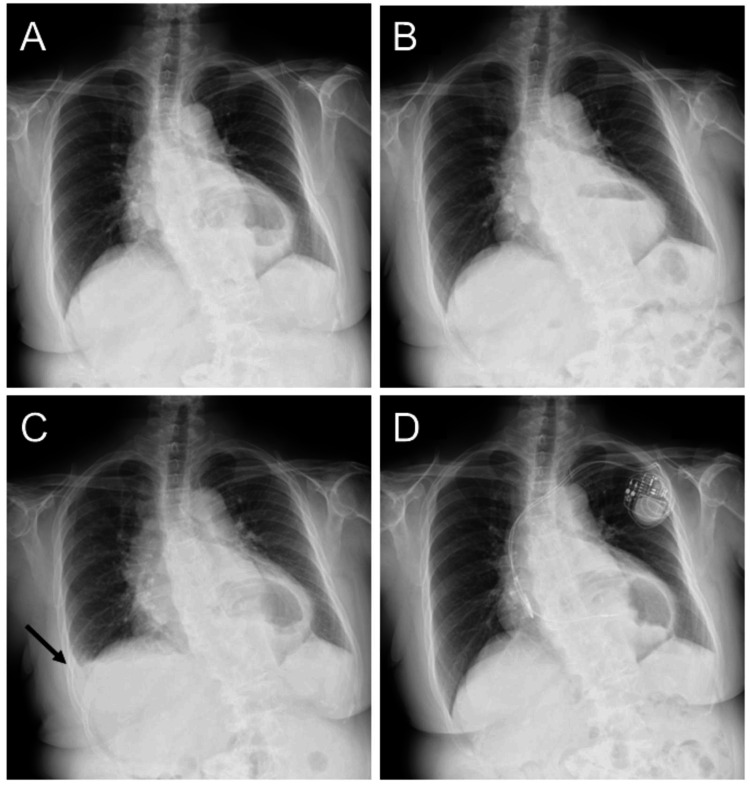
Serial chest radiographs The patient had a history of an asymptomatic hiatal hernia associated with scoliosis. Hiatal hernia had been incidentally found on chest radiographs three years before (A,B). Right-sided pleural effusion newly appeared (arrow), suggesting heart failure (C). Follow-up chest radiograph after pacemaker implantation showed complete resolution of right-sided pleural effusion with similar cardiac silhouette (D).
